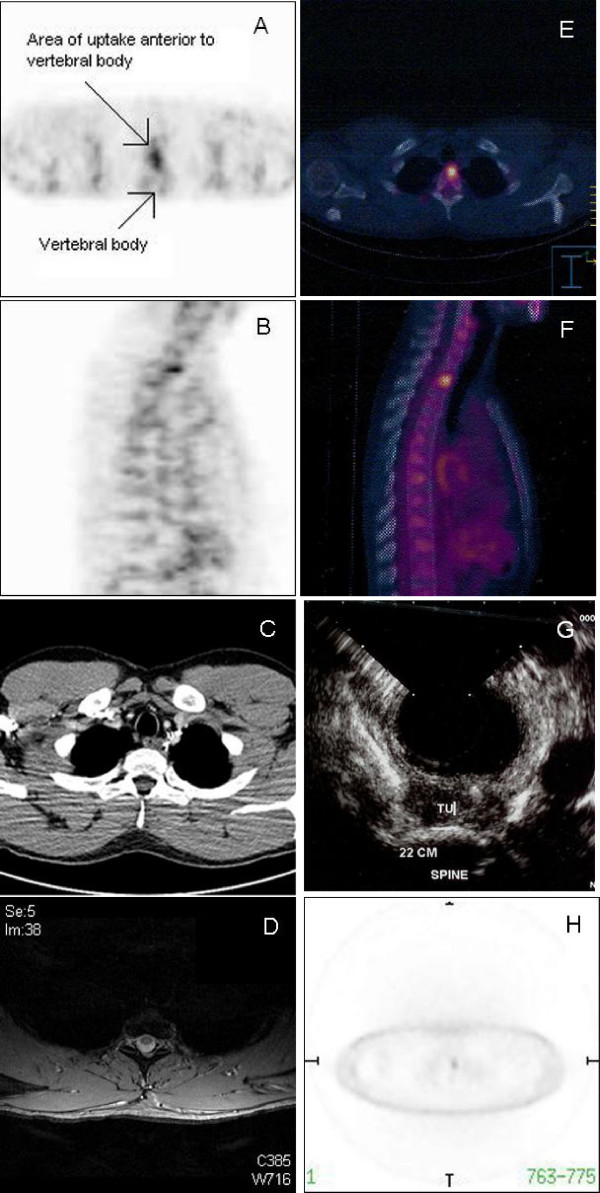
A & B: Axial and sagital view of ^18^FDG-PET scan of the patient showing region of ^18^FDG uptake anterior to the vertebral body. C: CT scan of the positive region identified on the ^18^FDG-PET scan. D: MRI of the thoracic spine in the region identified on the PET scan. E & F: Axial and sagital view of PET/CT scan of the patient showing that the lesion with ^18^FDG uptake was within the vertebral body. G: Endoscopic ultrasound image showing the tumour anterior to the vertebral body. H: Non-attenuated PET scan image from the PET/CT scan.
